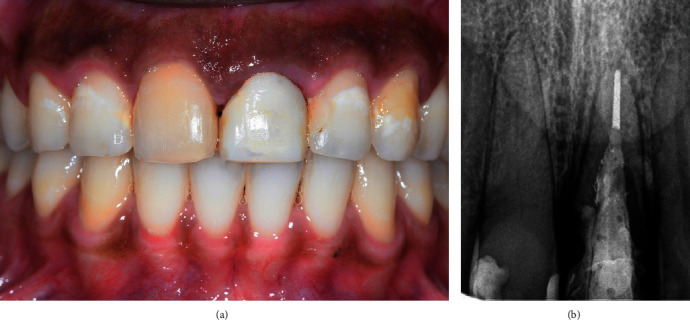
Clinical and radiographic presentation after orthodontic extrusion of tooth 21. (a) Intraoral frontal view showing the coronal relocation of the gingival margin and preservation of interdental papillae. (b) Periapical radiograph showing the coronal repositioning of the apex of the tooth and bone fill proximally.
